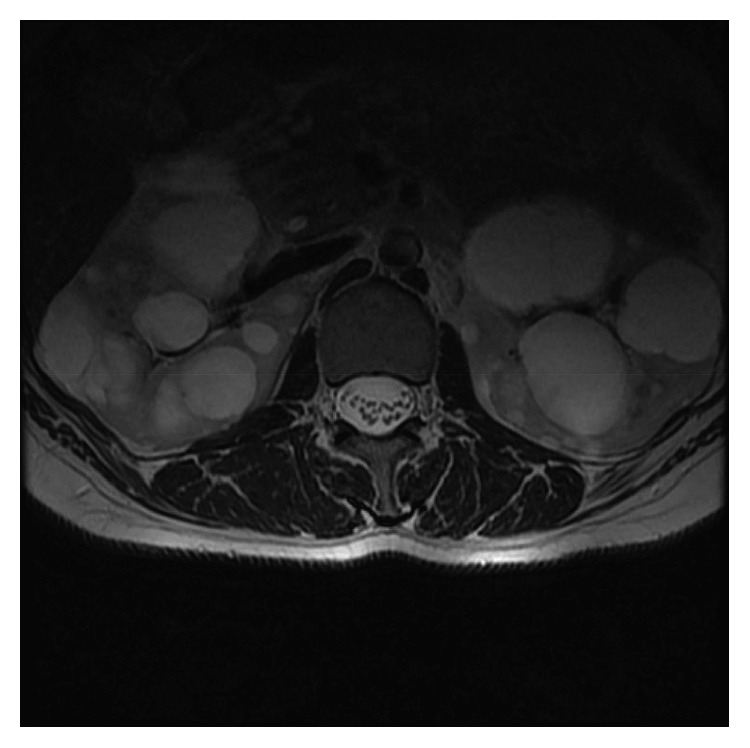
T2-weighted axial MRI scan showing polycystic kidneys.
